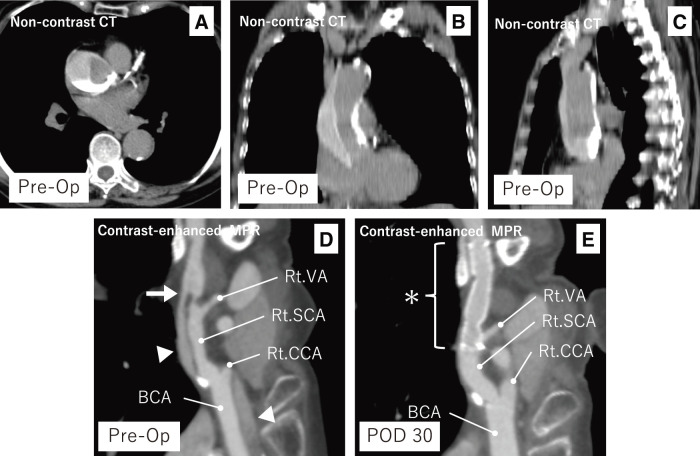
Fig. 2 Preoperative non-contrast CT (axial, coronal, and sagittal; A–C) and contrast-enhanced curved MPR images (preoperative day and POD 30; D, E). (A–C) Contrast pooling is visible along the posterior wall of the ascending aorta. (D) A focal intimal defect is seen in the distal right subclavian artery, with strong contrast enhancement at this level and gradual attenuation proximally. (E) The stent graft covers the entry site, and the false lumen has resolved. Arrow: entry; Arrowhead: false lumen; Asterisk: stent graft. VA: vertebral artery; SCA: subclavian artery; CCA: common carotid artery; BCA: brachiocephalic artery; MPR: multiplanar reconstruction; Op: operation; POD: postoperative day
